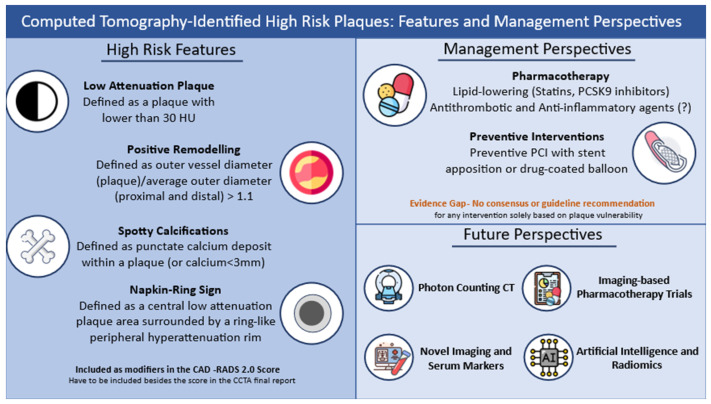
Computed tomography-identified high-risk plaques: features and management perspectives. Abbreviations: HU: Hounsfield Unit; CCTA; Coronary Computed Tomography Angiography; PCI: Percutaneous Coronary Intervention; CT: Computed Tomography.
